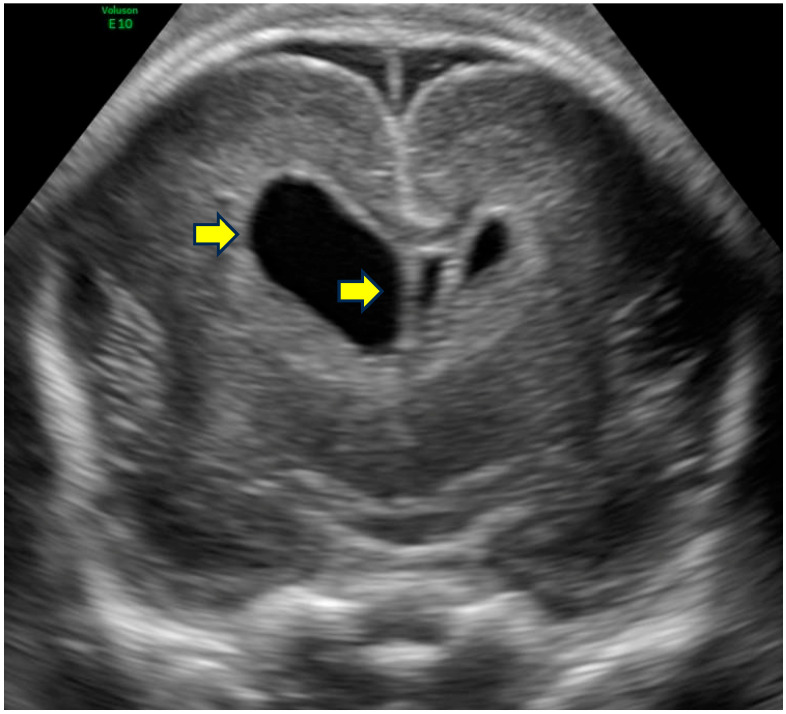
Coronal section showing asymmetry of the anterior horns and cavum septum pellucidum displacement. Fetal neurosonography at 22 weeks GA in a case with confirmed *TUBB3* variant. Arrows highlight dysmorphic and dilated frontal horns with displacement of the cavum septi pellucidi.
